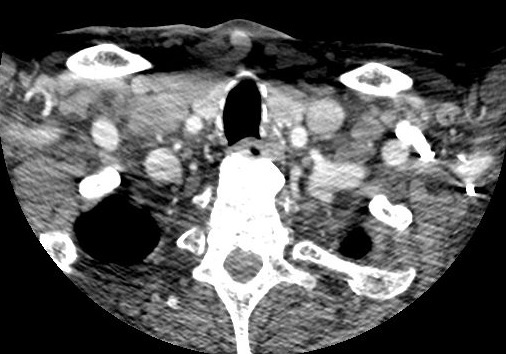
Neck computed tomographic scan showed osteoarthritic changes consistent of anterior cervical osteophytes causing displacement of the upper airway and compression of esophagus
